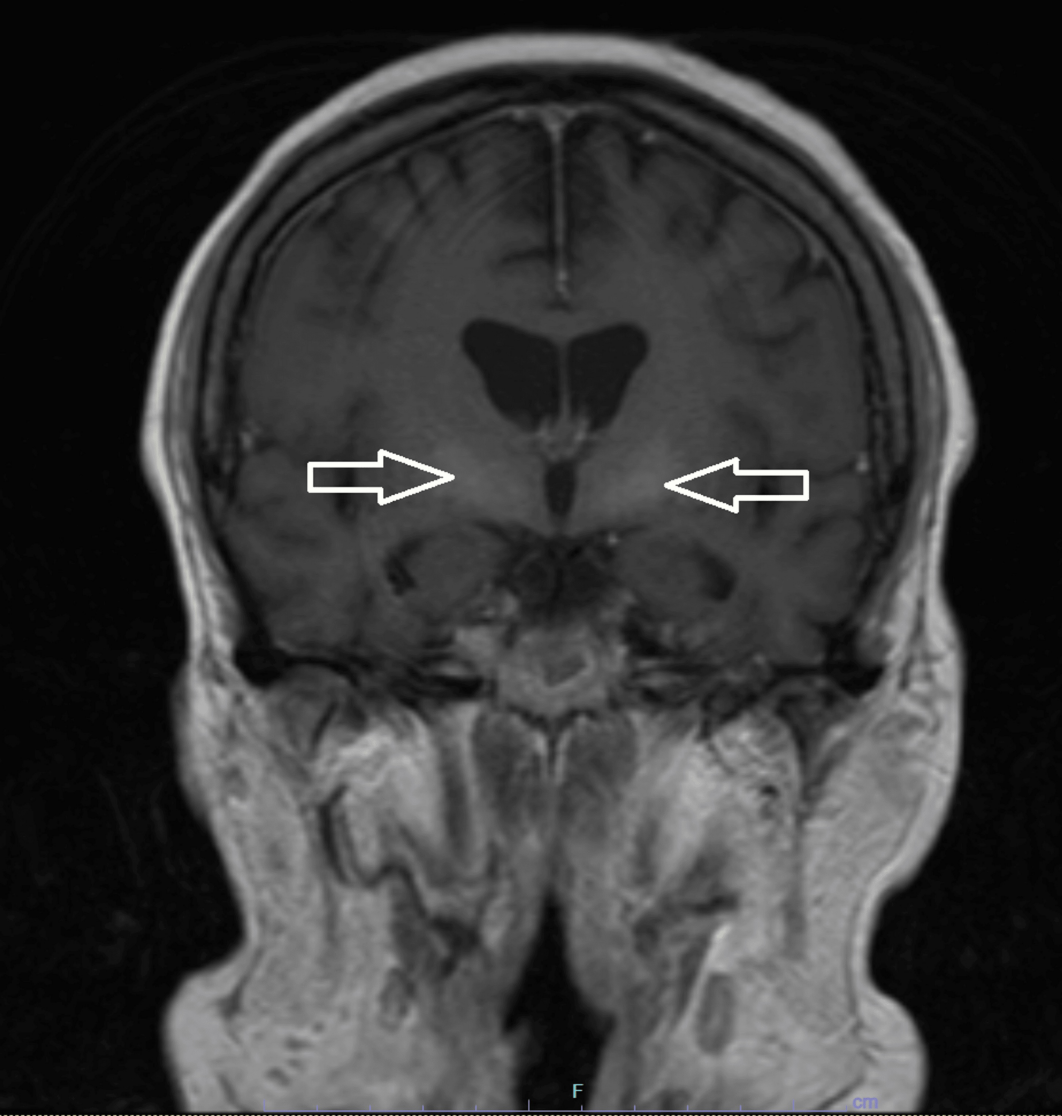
Coronal T1-weighted MRI of the brain, with the arrows marking the globus pallidus hyperintensity bilaterally
